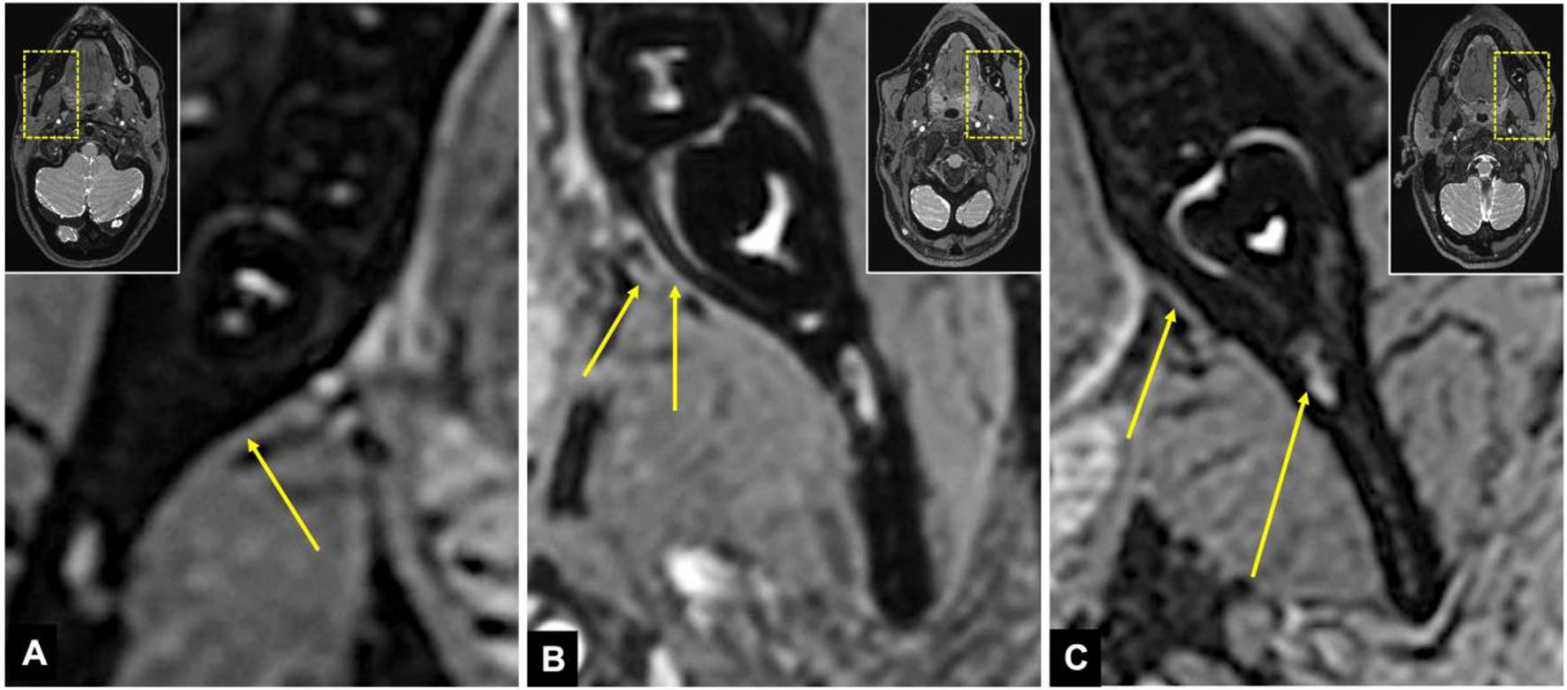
Visualization of the lingual nerve in the mandibular third molar region in axial 3D double-echo steady-state (3D-DESS) MRI reconstructions: a The arrow indicates the hyperintense signal intensity of the lingual nerve as it enters the mandibular third molar region; b The shorter arrow visualizes the main branch of the lingual nerve in the mandibular third molar region, while the longer arrow represents the branch described in the literature as the "gingival branch of the lingual nerve" or "collateral nerve twig"; c another example where the shorter arrow represents the lingual nerve while the longer arrow visualizes the inferior alveolar nerve
